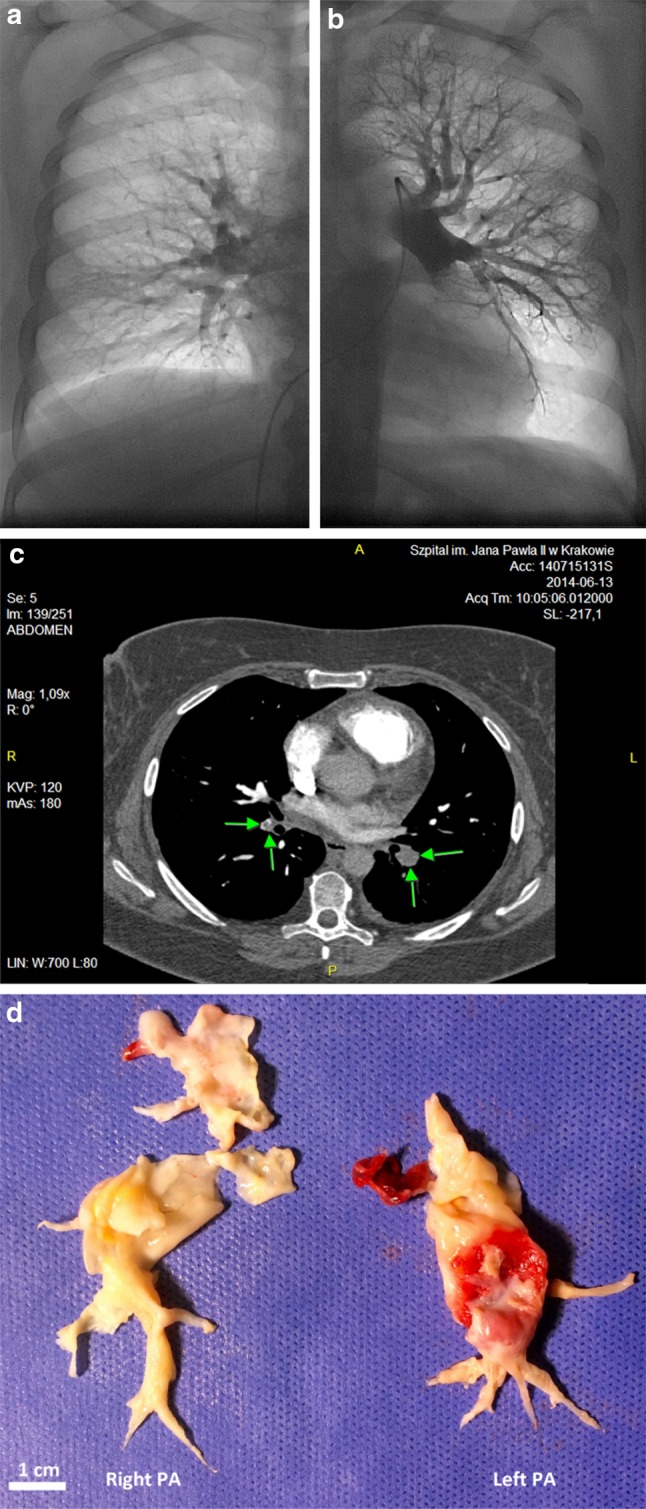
Pulmonary angiogram, computed tomography and intraoperative images. Pulmonary right (a) and left (b) angiogram showing deficient perfusion, more pronounced in the right pulmonary artery (a). Contrast-enhanced computed tomography showing thrombotic material in lower-lobe branches of right and left pulmonary arteries (c). Intraoperative picture of the thrombotic material removed from pulmonary arteries during PEA (d), *scale bar* 1 cm
